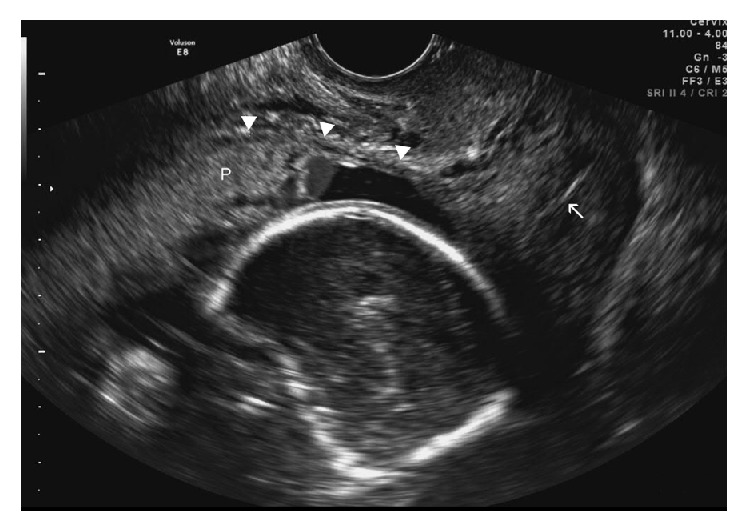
Sagittal transvaginal sonogram performed 3 months later shows intrauterine pregnancy with 19-week sized fetus. Thinned lower anterior uterine wall (arrowheads) and contiguous placenta (P) are suspicious for placenta accreta (arrow: cervical canal).
